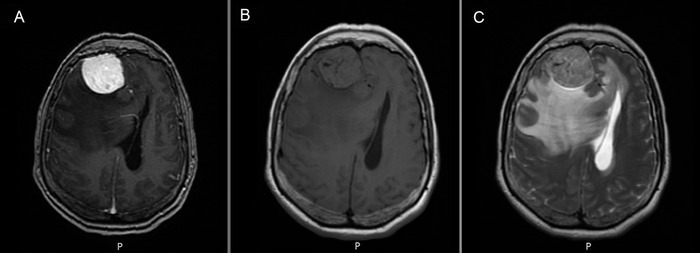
(A) T1 plus contrast, (B) T1, and (C) T2-weighted magnetic resonance axial images of the brain taken during the patient's presentation to the emergency department show a large right frontal-enhancing, well-circumscribed, extra-axial mass measuring 4.0 × 3.9 × 4.2 cm, resulting in mass effect and vasogenic edema within the right frontal lobe with an associated 2.1-cm leftward midline shift and right-sided herniation.
